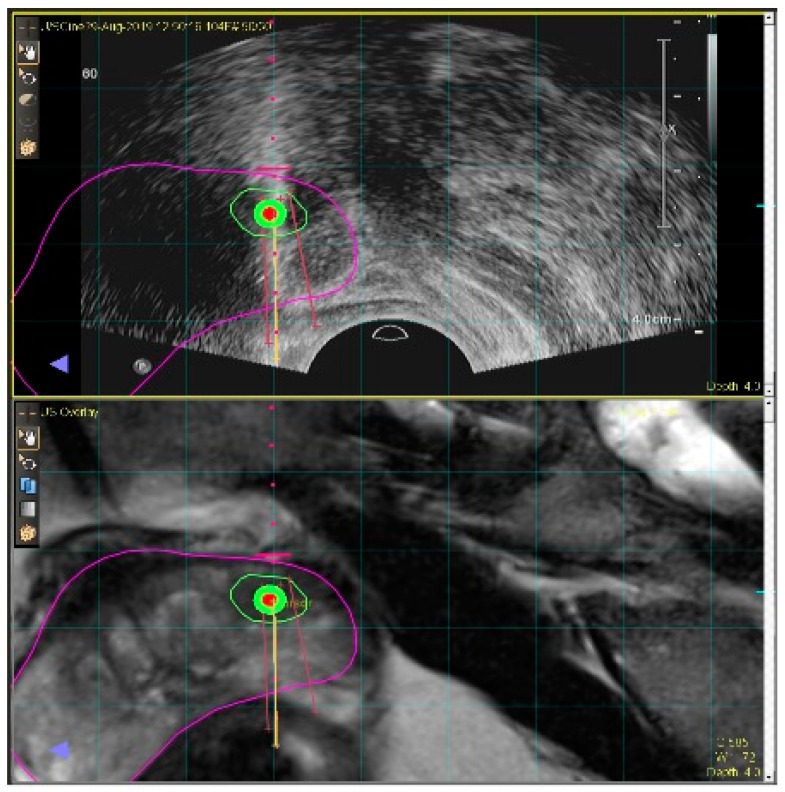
MRI–US fusion technique for targeted prostate biopsy requires precise registration between pre-operative prostate MRI (bottom image) and real-time ultrasound (top image).
